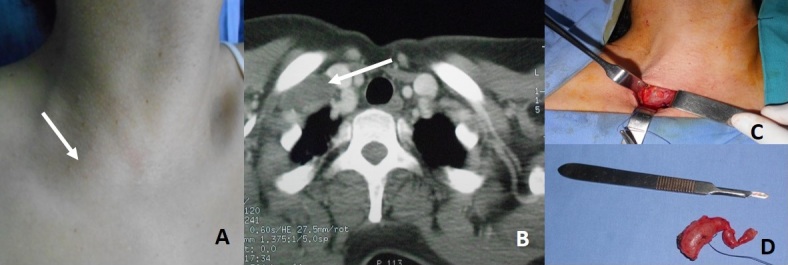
A) right supraclavicular mass on examination (arrow); (B) CT scan of the neck, showing a well-defined supraclavicular cystic mass (arrow); (C) Intra-operatively the mass appeared fluid filled and lobulated; (D) Excision of the cystic mass
